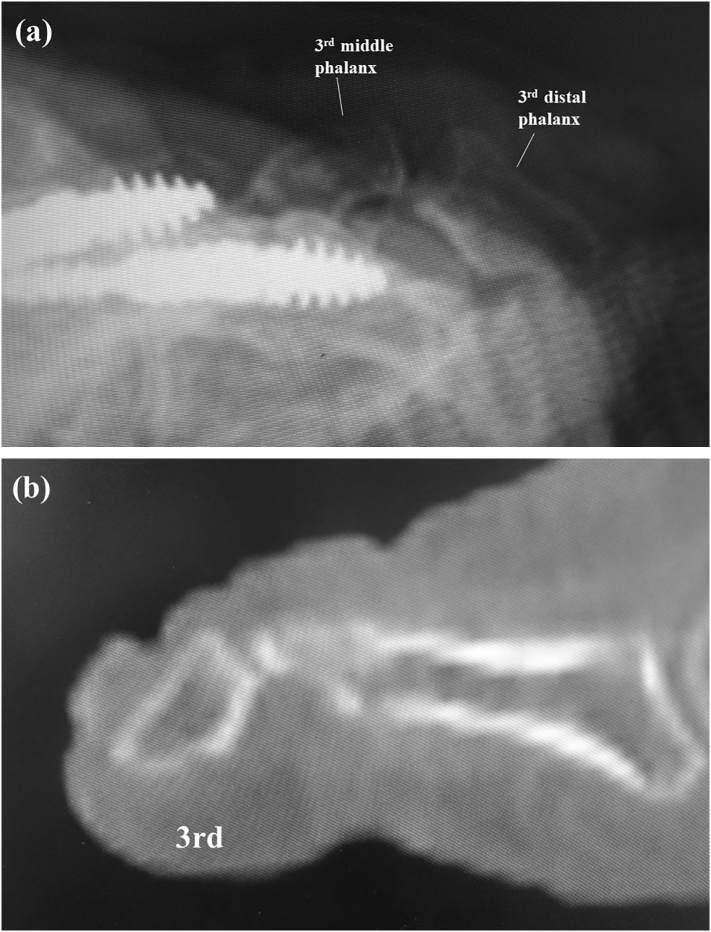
A lateral radiograph (a) and a sagittal computed tomography scan (b) show decreased hyperflexion of the distal interphalangeal joint.
